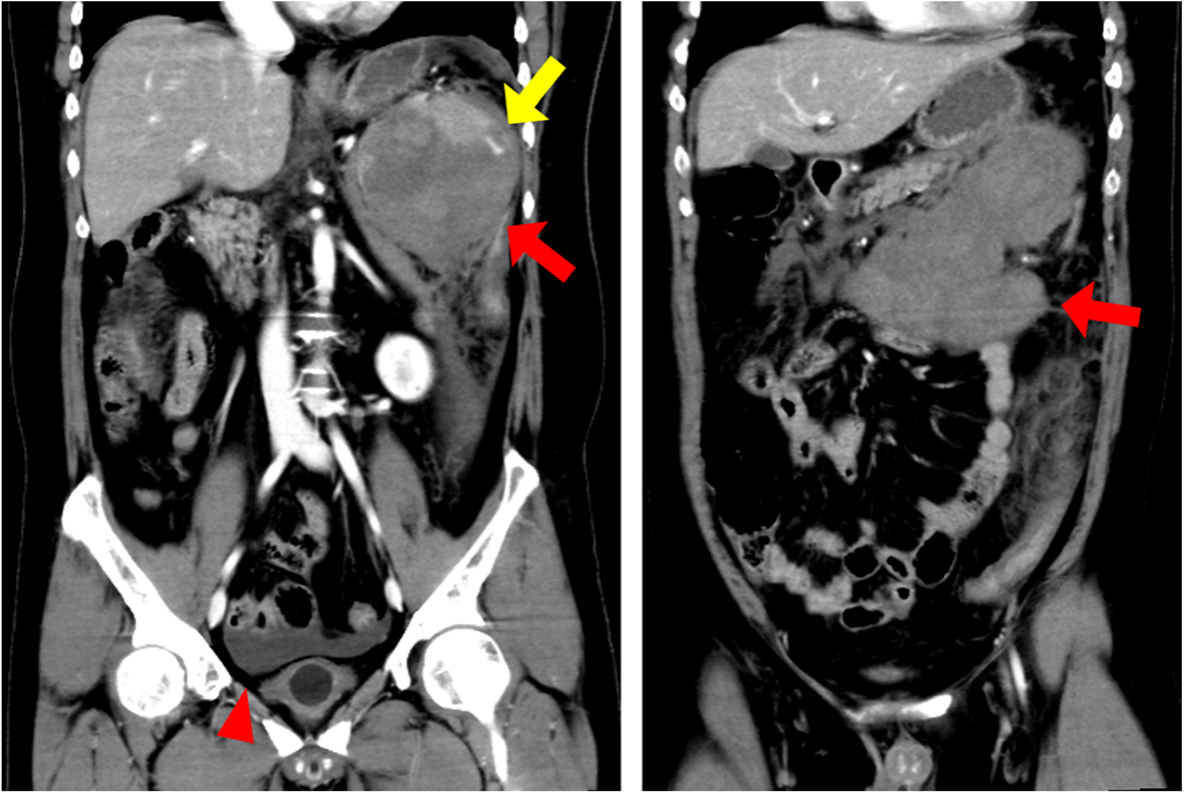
Images of frontal sections of contrast-enhanced computed tomography at a portal phase. *Red arrows* show heterogeneously enhanced abdominal mass which ranges from an intramesenteric space of the transverse colon to the left upper abdomen with extension to 25 cm in the longest diameter. A *yellow arrow* shows extravasation of the contrast material into the mass. An *arrowhead* shows ascites in Douglas’ pouch
